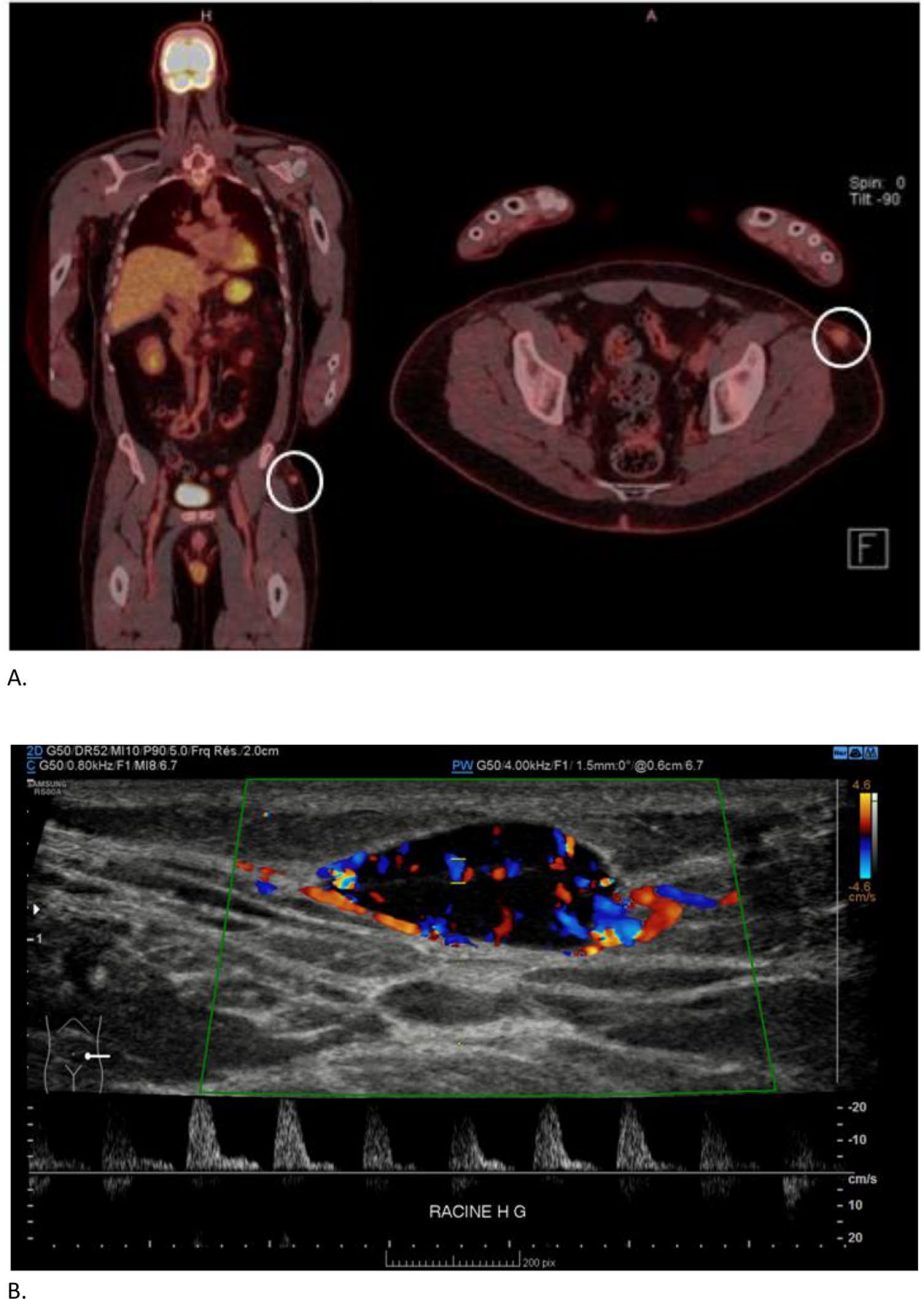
The tumor on the PET-CT and at the ultrasound scan. A Nodular subcutaneous hypermetabolic (max SUV 3) lesion in the left thigh. B Subcutaneous hypoechogenous lesion at the ultrasound measuring 17 × 8 × 14mm, vascularized with arterial and venous flow with Doppler
